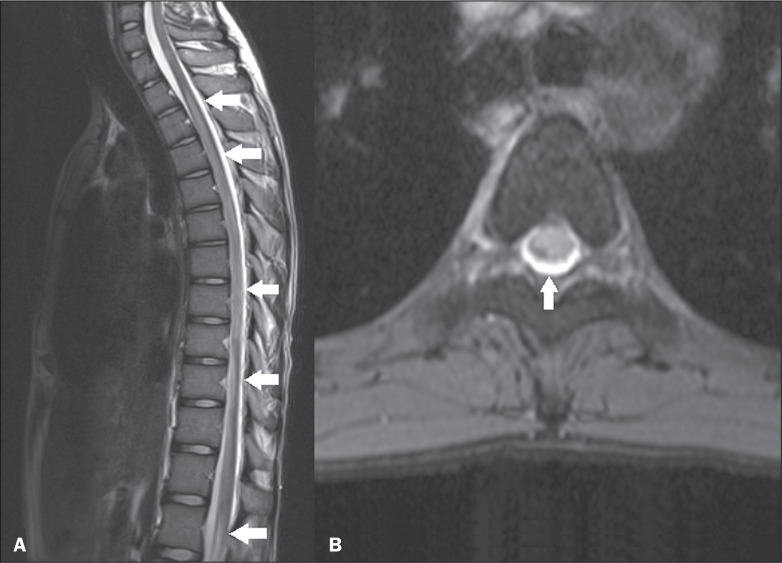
Acute disseminated encephalomyelitis. Sagittal T2-weighted and axial
